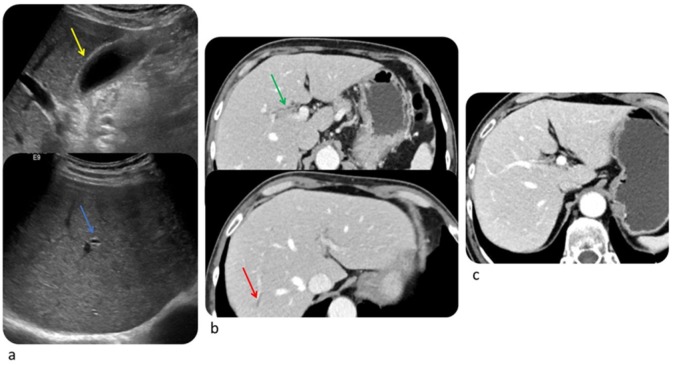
Immune related cholangitis in a 69-year-old female with metastatic melanoma treated with ipilimumab + pembrolizumab. Ultrasound shows layered gallbladder wall thickening likely representing gallbladder wall edema (a, yellow arrow) and hyperechogenicity of periportal space (a, blue arrows). Computed Tomography image shows mild wall thickness and contrast enhancement of the right hepatic duct (b, green arrow) and mild dilated intrahepatic bile duct (b, red arrow). Computed Tomography performed after steroid therapy demonstrates resolution (c). © Department of Radiology, Candiolo Cancer Institute—IRCCS. Turin.
